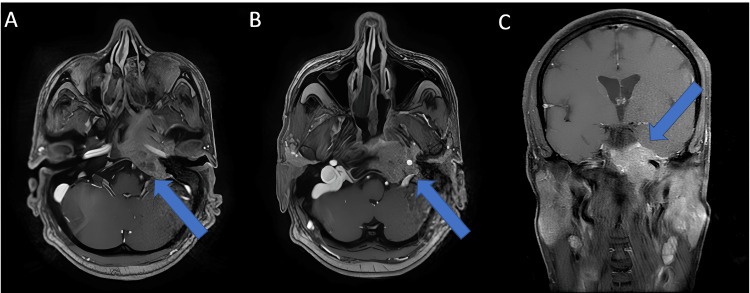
Angio-MRI of the nasopharynx in contrast-enhanced T1 sequence A: axial view showing a soft-tissue lesion (arrow) centered on the left posterosuperior wall of the nasopharynx, measuring approximately 53 × 42 mm, invading the parapharyngeal space and the left infratemporal fossa; B: axial view showing the lesion (arrow) encasing the left internal carotid artery; C: coronal view demonstrating the intracranial extension of the tumor (arrow).
